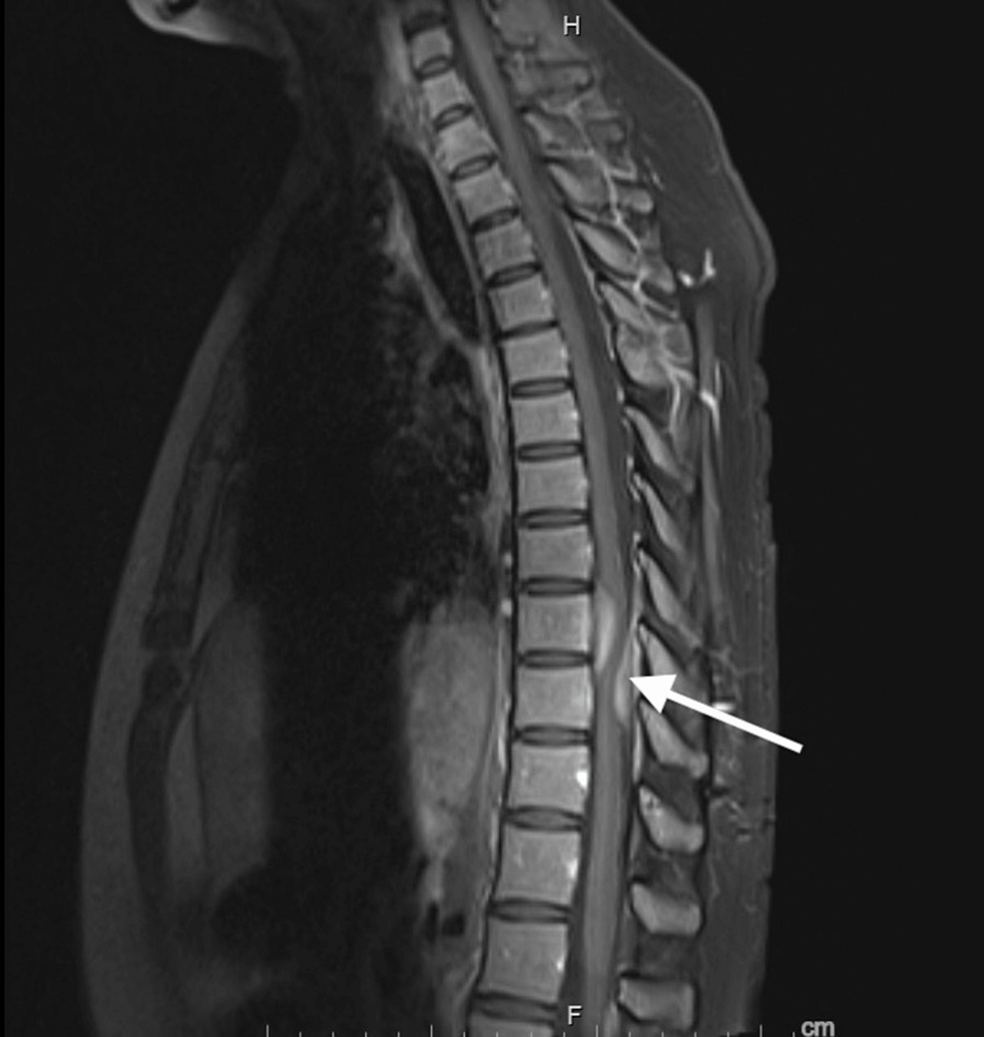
A surveillance magnetic resonance imaging (MRI) of the thoracic spine. A follow-up MRI of the thoracic spine showing relative stability/slight size improvement of the thoracic lesions coinciding with clinical improvement in response to repeated courses of steroids (arrow).
